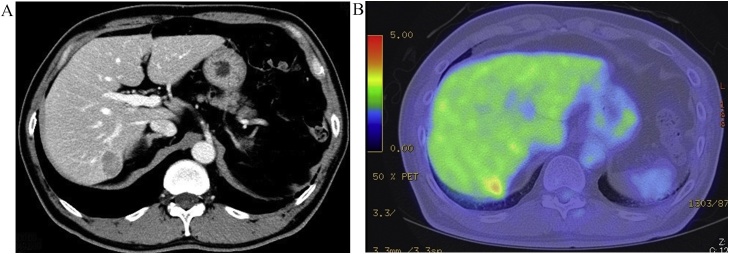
A) Abdominal CT showing a mass measuring 27 mm in diameter in S7 of the liver. B) FDG-PET showing high accumulation of FDG with an SUVmax 3.4 in the liver tumor.
